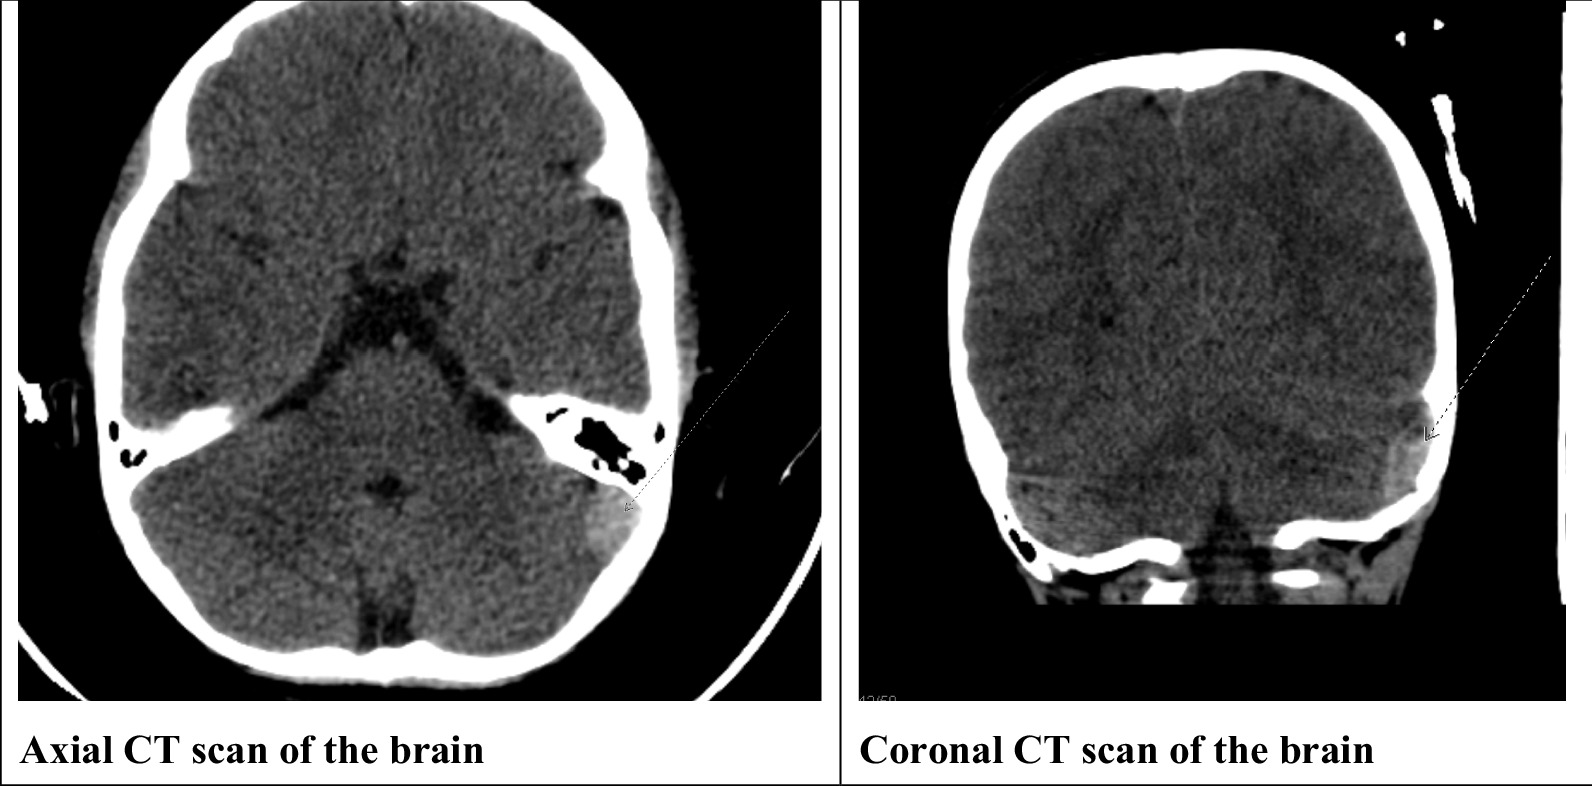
A plain axial CT scan of the brain showing mild interval regression of the left-sided posterior fossa/cerebellar epidural hematoma
